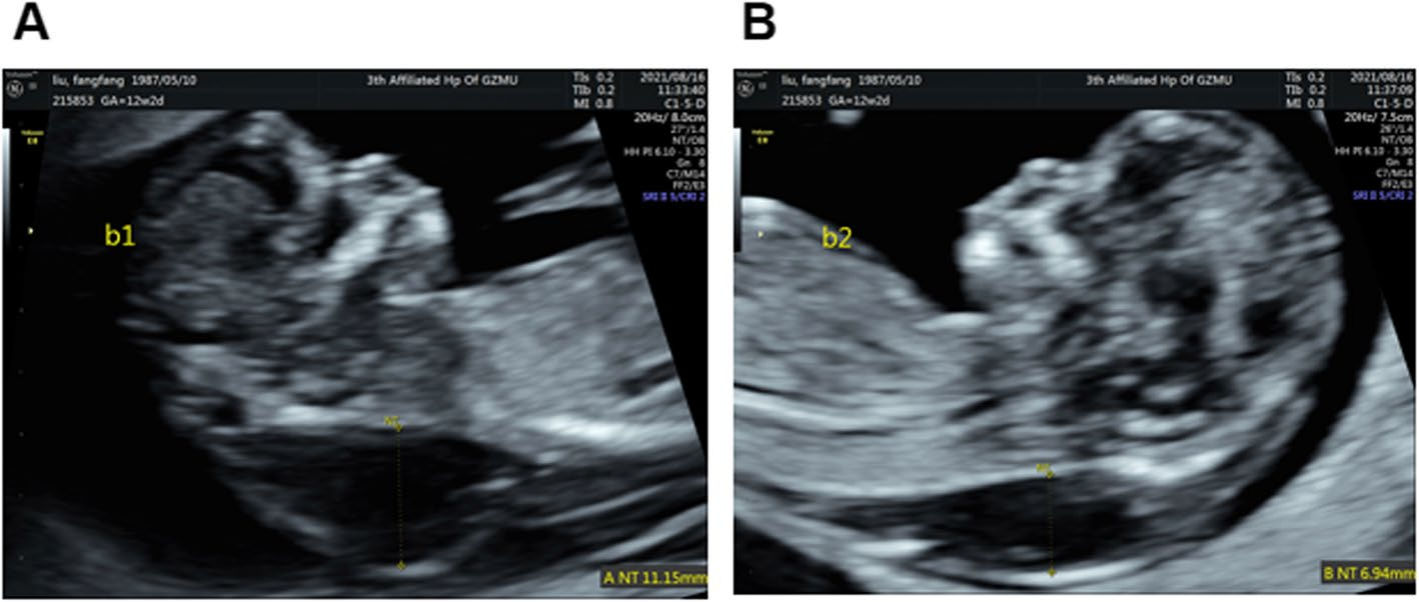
Ultrasonic image of twin fetal NT. A Ultrasonic image of fetal 1 NT. B Ultrasonic image of fetal 2 NT
